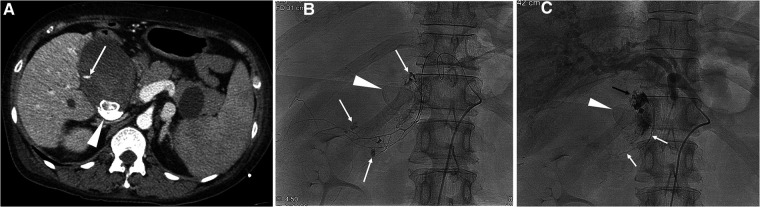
A 49-year-old female with liver cirrhosis and hemorrhagic cholecystitis. (A) CT scan shows extravasation of contrast media (arrow) in the gallbladder. Note gall stones (arrowhead). (B) Selective angiogram of the cystic artery shows multifocal extravasation (arrows) of contrast media. Note gall stones (arrowhead). A mixture of n-butyl cyanoacrylate and Lipiodol was used for embolization of the main cystic artery. (C) The final angiogram shows cessation of active bleeding. Note glue material in the cystic artery (white arrows) and in the lumen of the gallbladder (black arrow), and gall stones (arrowhead). Development of ischemic cholecystitis occurred after embolization of the cystic artery, resulting in sepsis and multiorgan failure. The patient died three days after cystic artery embolization.
